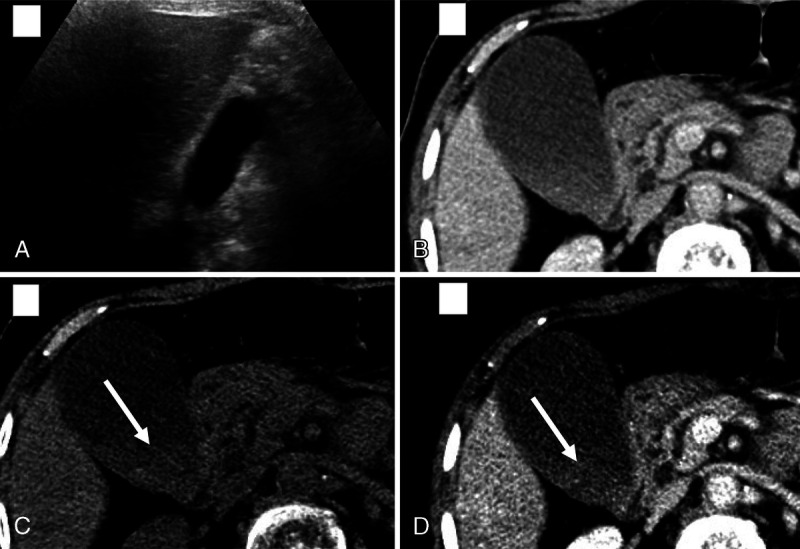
False positive: This was called as positive for GSs by all 4 reviewers based on the VNC (C) and 50-keV VMC (D) images. The PC image (B) was equivocal. Ultrasound performed (A) the same day showed no GSs.
